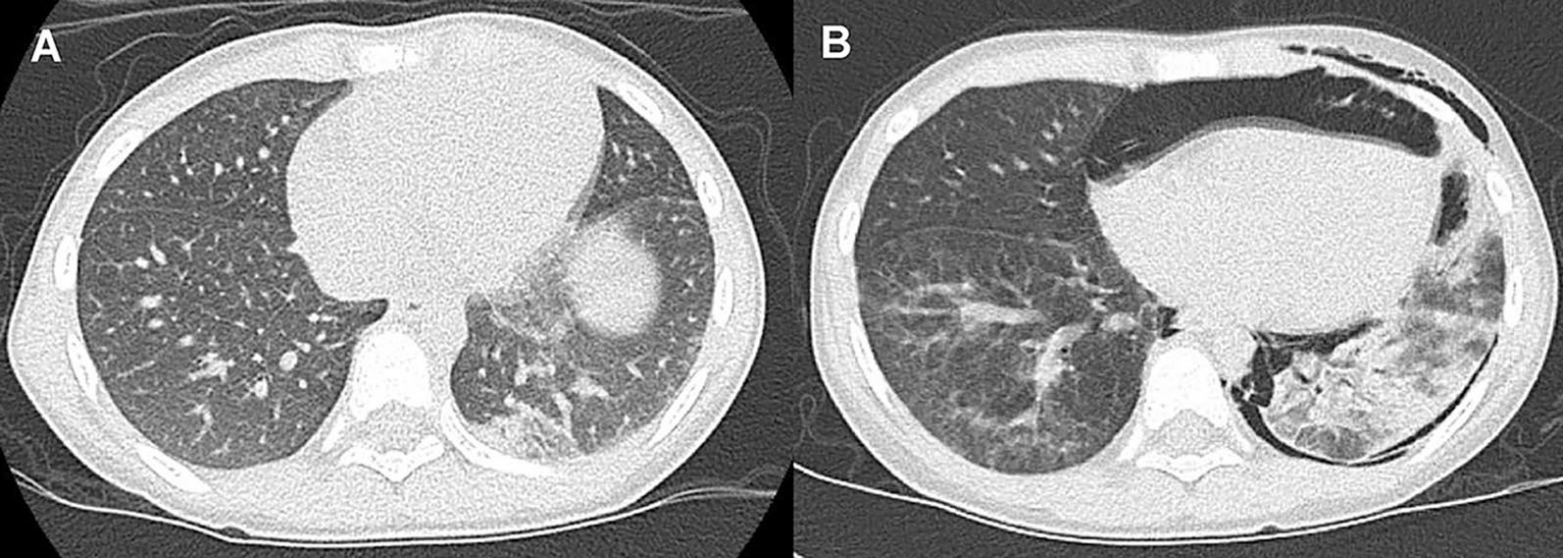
Rapidly progressive interstitial lung disease (RP-ILD) in dermatomyositis. A 5-year-old girl with positive anti-MDA5 antibody. A The initial CT showed a pleural based GGO in the left lung base. B Six weeks later, she developed respiratory failure. GGOs extended into the wide zones of both lung bases, and complicated by pneumothorax, pneumomediastinum, and subcutaneous emphysema
